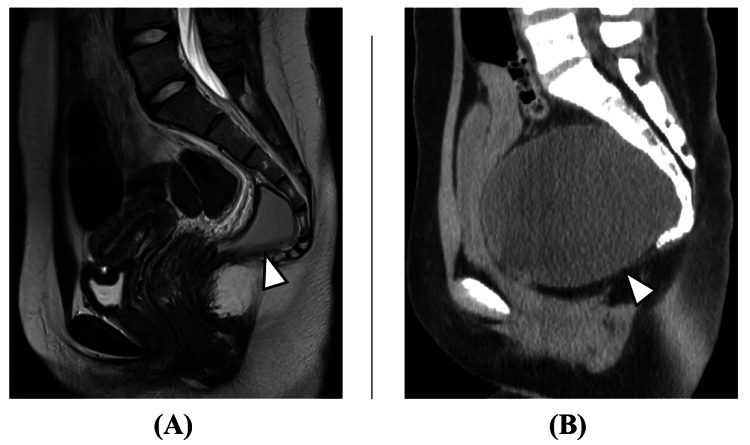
Preoperative pelvic imaging. (A) Immediately after aspiration (MRI T2 sagittal). (B) Three weeks after aspiration (CT sagittal). Approximately 500 ml of aspirated fluid had refilled within three weeks (arrowheads).
